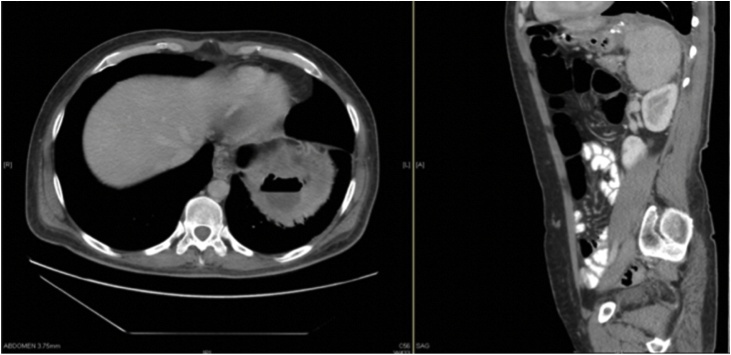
Axial and Sagittal CT view showing a left subphrenic collection showing an air-fluid level associated with mild thickening of the diaphragmatic crus and linear atelectatic changes of the adjacent lung.
